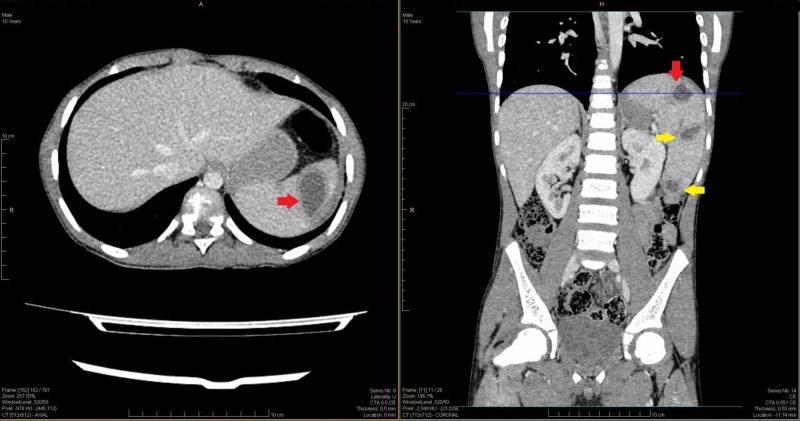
Axial and coronal view of abdominal CT scan showing multiple hypo-dense areas of hydatid cysts (red and yellow arrows), largest cyst (red arrow). CT: Computed tomography
